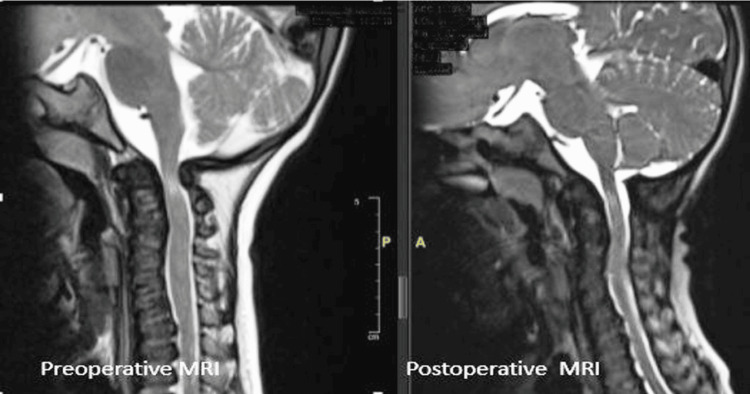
Case 1: Preoperative and postoperative MRI T2WI showing cervicomedullary compression with hypoplasia of the odontoid peg, and postoperative decompression of the spinal cord following surgery. T2WI: T2-Weighted Imaging.
